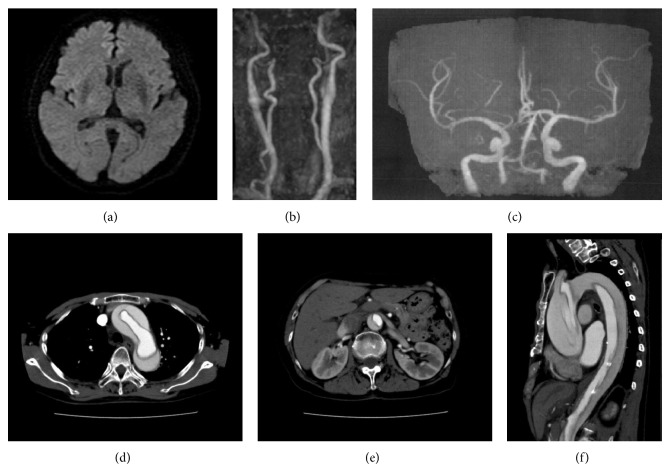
Axial head MRI-diffusion-weighted image (DWI) (a), carotid MR angiography (b), head MR angiography (c), axial chest to abdominal enhanced CT ((d), (e)), and sagittal view (f).
